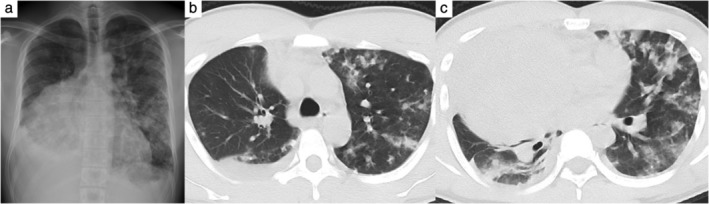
(a) Chest radiography in a patient with dyspnea and a high fever showed multiple mottled shadows in the bilateral lung. (b and c) Computed tomography showed multiple mottled shadows and pleural effusion in the bilateral lung.
